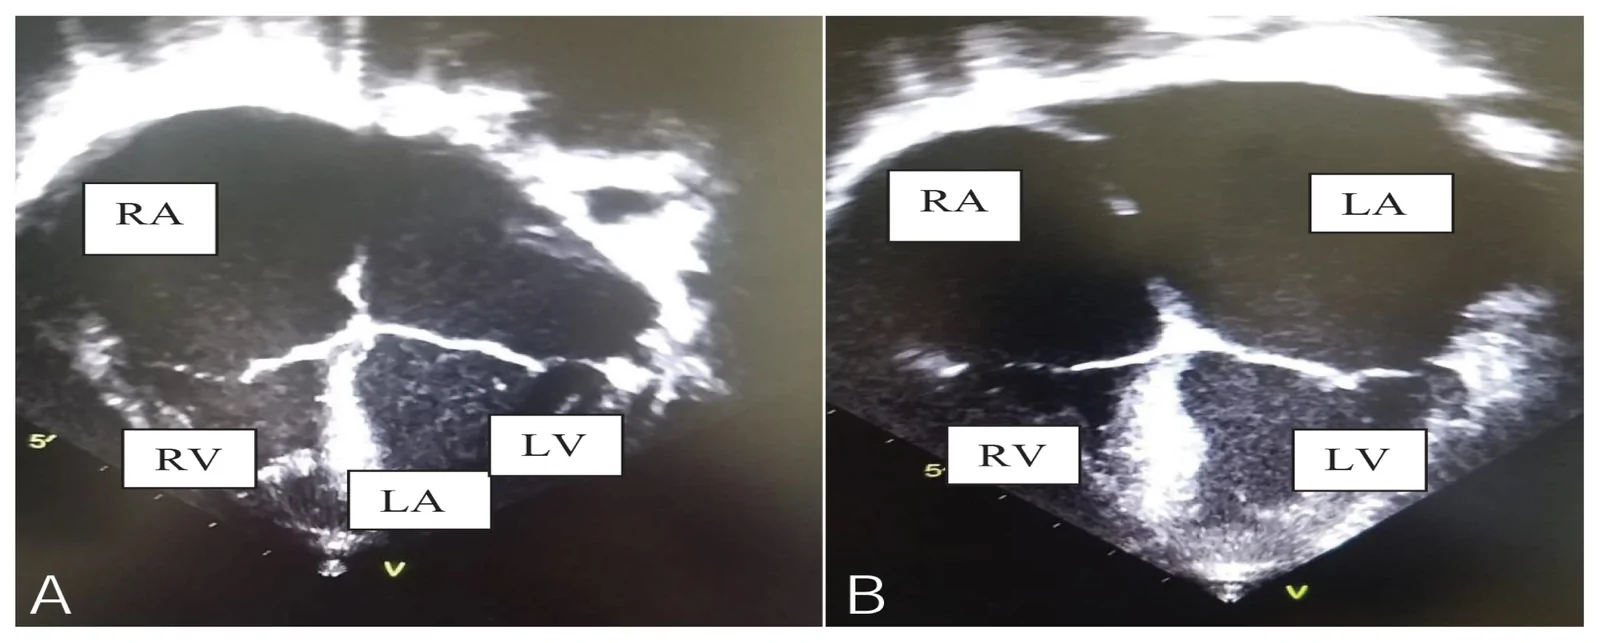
Echocardiography of (A) case 1 and (B) case 2 that presented to a tertiary care hospital in Muscat, Oman in 2019. RA = right atrium; LA = left atrium; RV = right ventricle; LV = left ventricle.
